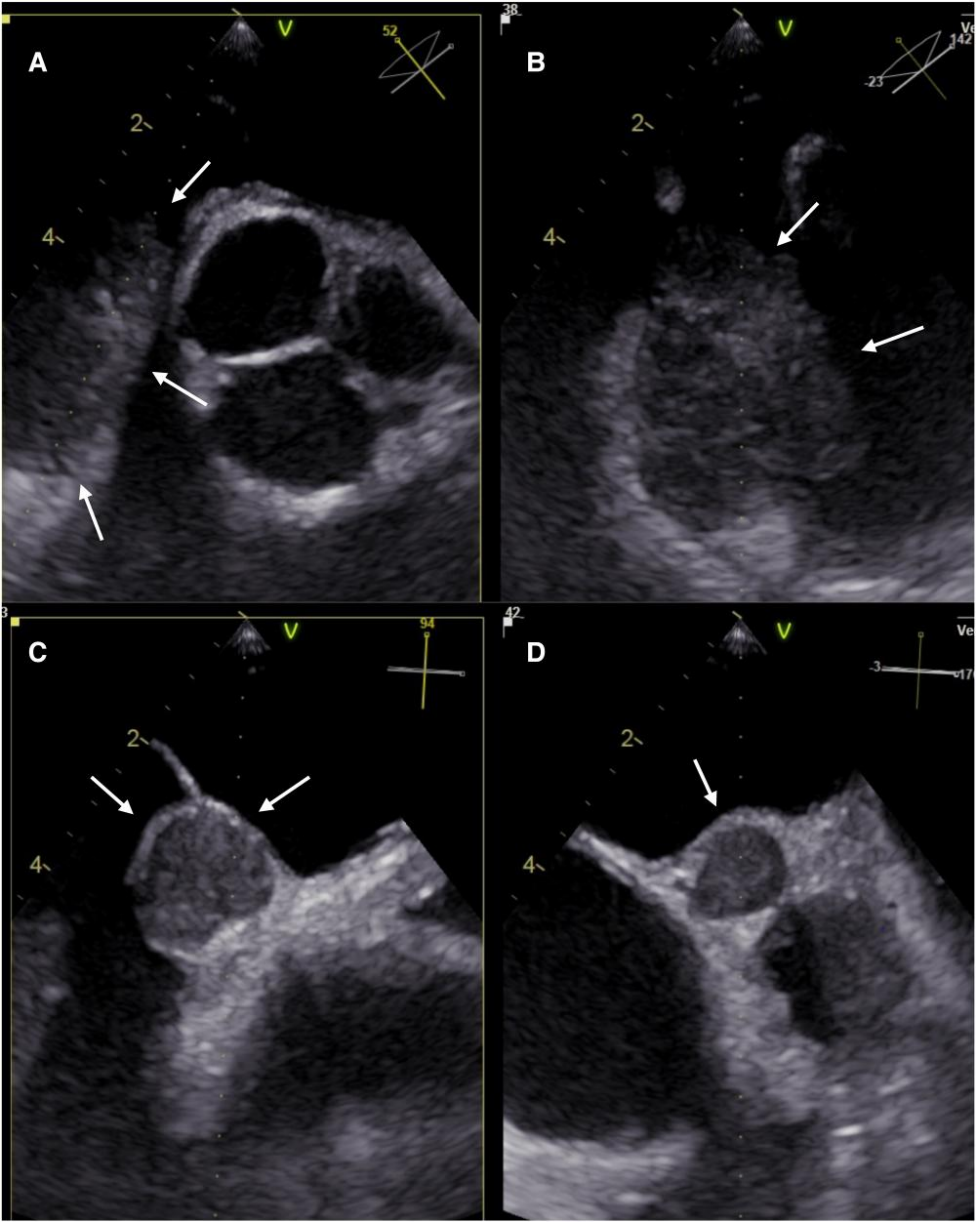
(*A*) Transoesophageal echocardiogram at 52° at the level of the aortic valve demonstrates the cardiac mass in the area of the right atrium (arrows) (*B*) corresponding orthogonal plane in the multi-plane echocardiogram. (*C*) Transoesophageal echocardiogram at 94° demonstrates second encapsulated cardiac mass antero-superior to the atrial septum (*D*) corresponding orthogonal plane in the multi-plane echocardiogram.
